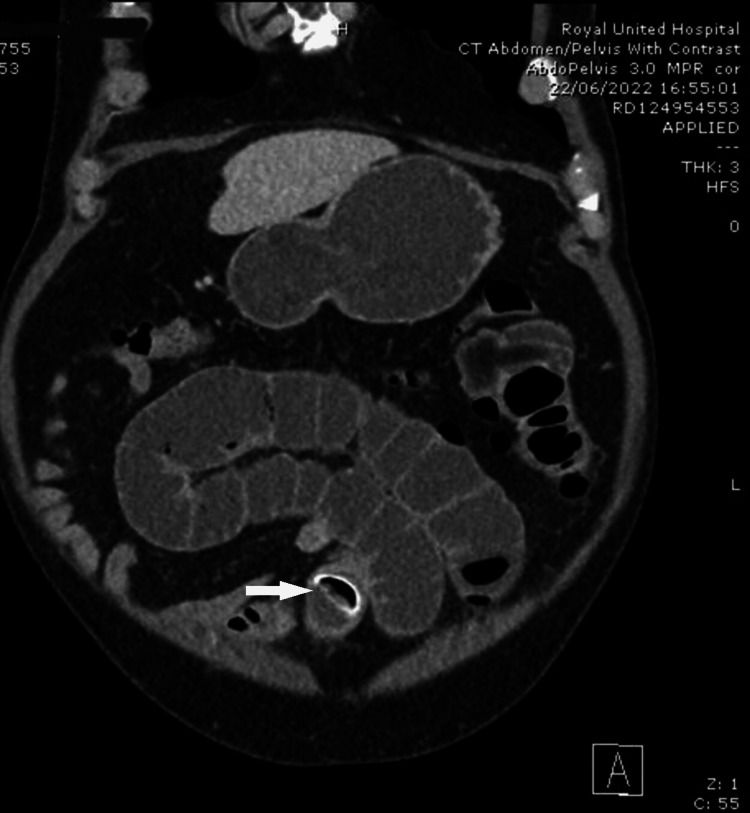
Computed tomography image White arrow showing intraluminal foreign body causing small bowel obstruction
